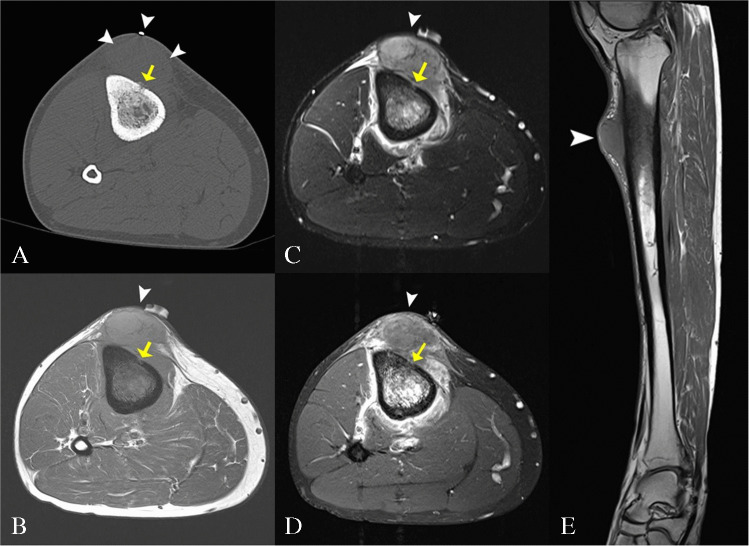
33-year-old male with Ewing sarcoma of the proximal tibia. Axial CT (A) and axial T1 (B), STIR (C), and T1 FS post contrast (D) and sagittal T1 (E) MRI images demonstrating low to intermediate T1 signal, variable intermediate to high T2 signal, and heterogeneous enhancement with a linear lower-attenuation/intermediate signal intensity channel extending through the low signal intensity cortex (arrows) allowing communication between the intraosseous and soft tissue components with an adjacent associated soft tissue mass (arrowheads)
